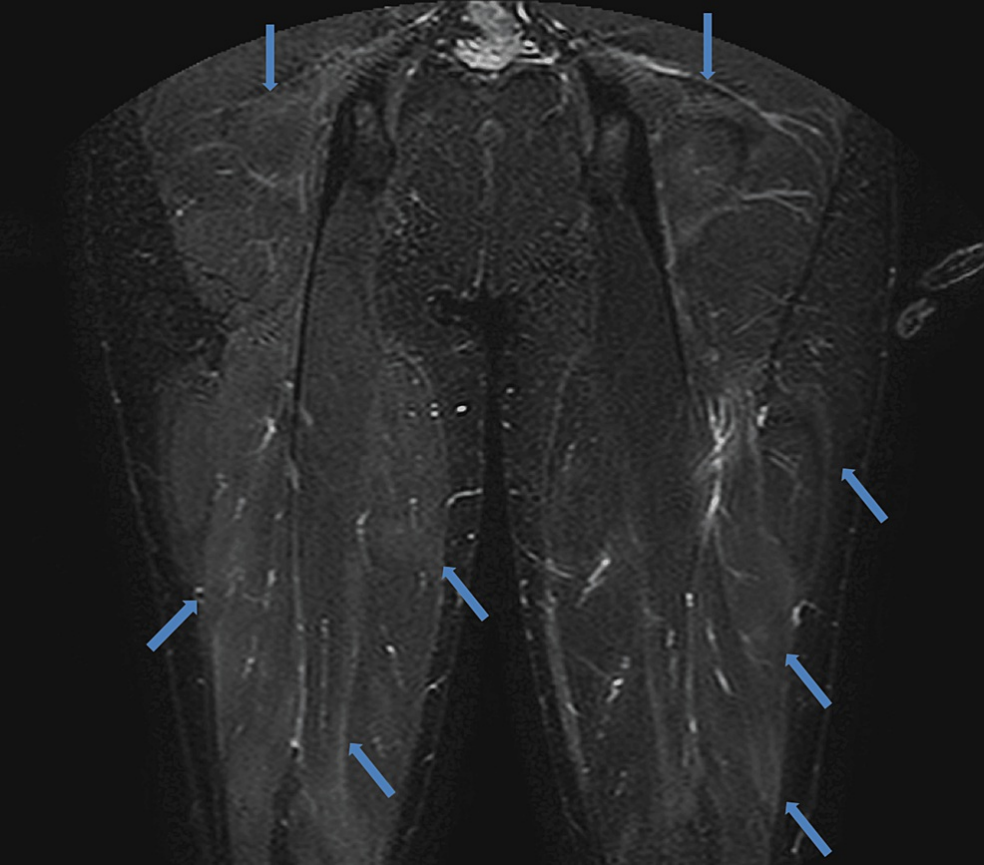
Magnetic resonance imaging short TI inversion recovery of the thighs (anteroposterior view) Magnetic resonance imaging short TI inversion recovery of the thighs (anteroposterior view) showing weak, heterogeneous high signal in the fascia and the muscles of gluteus, abductor, adductor, quadriceps, and hamstring muscles of both thighs (blue arrows).
